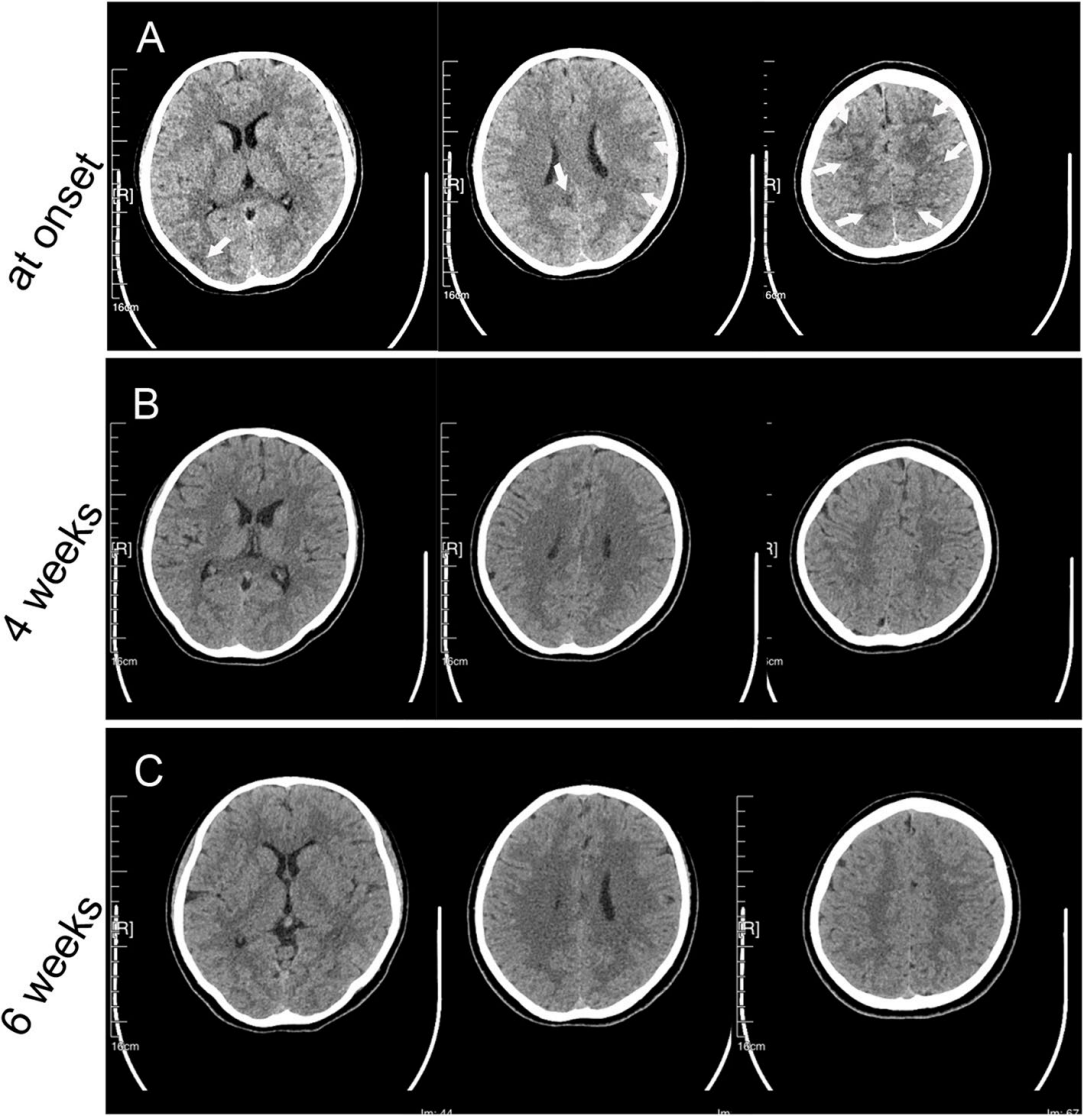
Initial and follow-up brain CT of the patient. Brain CT images at the onset of the patient’s seizure show hypointense signals in the bilateral frontal, parietal and occipital lobes (A, white arrow). Follow-up CT images at 4 weeks (B) and 6 weeks (C) after the patient’s seizure both show resolution of the hypointense lesions
